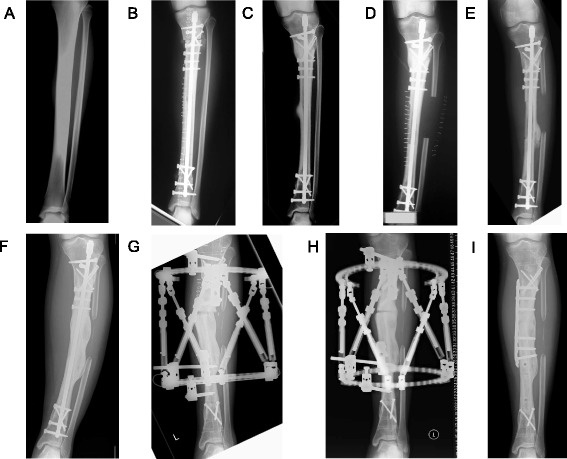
Pre- and postsurgical radiography of a patient with tibial osteosarcoma. a Anteroposterior radiograph taken at admission revealed an osteoblastic lesion on the left tibia. b Radiograph taken after the first surgery reveals that the frozen autograft was fixed and aligned well. c Radiograph taken 17 months after the first surgery shows a recurrent lesion on the surface of the frozen autograft. d Radiograph after the second surgery. e Radiograph taken 10 months after the second surgery shows a varus deformity with callus formation in the frozen autograft. Bone union with the grafted fibula had not been achieved. f Radiograph taken 5 years after the second surgery shows a varus deformity (19°), although union with the grafted fibula had been achieved. g Radiograph taken after the third surgery shows the osteotomy site in the frozen autograft and the partial correction of the deformity using the Taylor Spatial Frame. h Radiograph taken 9 months after the third surgery shows complete correction of the deformity and partial union of the osteotomy sites. i Radiograph taken after the fourth surgery shows fixation of the osteotomy site with a locking plate
